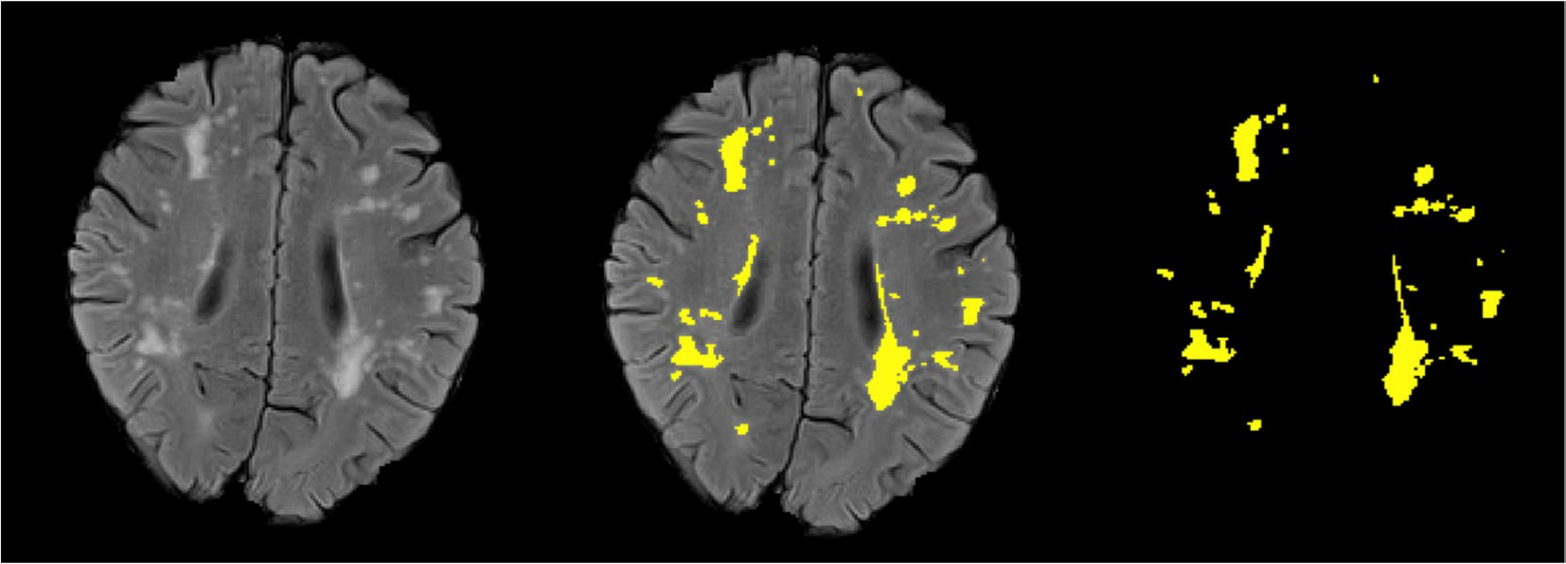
Example of a manual segmentation on a FLAIR image
